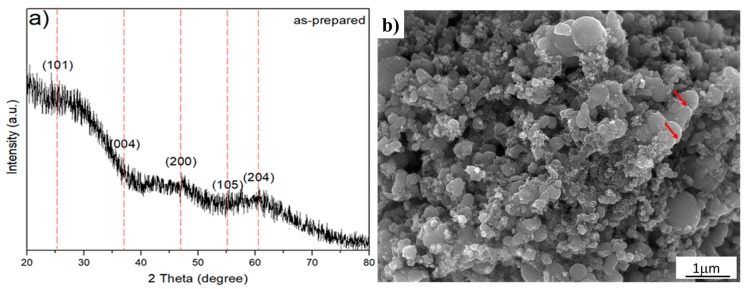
As-prepared sample. (a) Nanocrystalline TiO_2_ XRD pattern of the sample synthesized by ultrasonic radiation for 50 min, whereby the dashed lines correspond strong diffraction peaks according to JCPDS card No. 21-1272 (anatase TiO_2_); (b) Typical FESEM image depicting the cavitation effect by sonochemical means.
